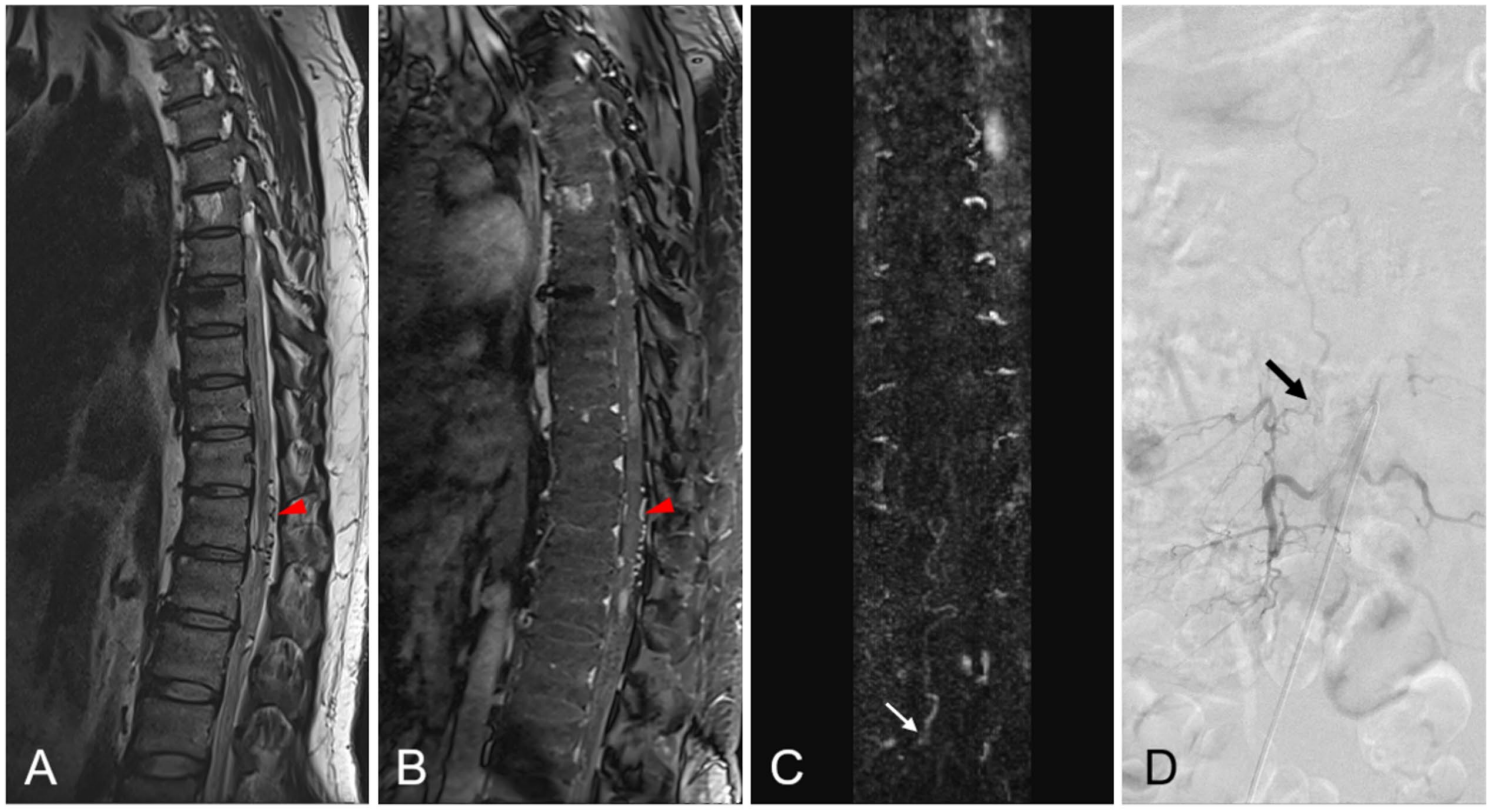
A 42-year-old patient with spinal dural arteriovenous fistula (SDAVF) at the L2-3 level on the right side. (A,B) Sagittal T2-weighted image and enhanced T1-weighted image shows prominent perimedullary vein (*red arrowhead*). (C) The coronal MRA MIP image demonstrates the feeding artery of the SDAVF at the L2-3 level on the right side (*white arrow*). (D) The localization of this origin is confirmed by DSA (*black arrow*).
